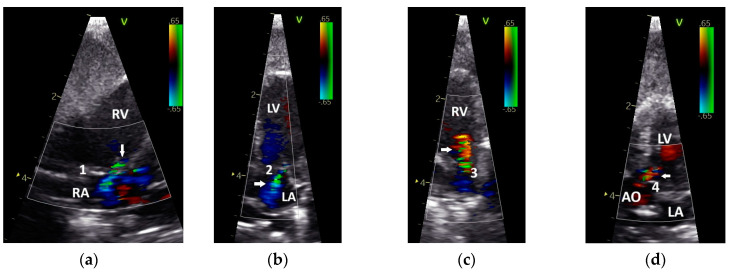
Insufficiencies of the right (a) and left (b) atrioventricular valve and the pulmonary (c) and aortic valve (d) of Congo grey parrots, visualized by colour flow Doppler echocardiography. Arrow: marked insufficiency jet; RV: right ventricle; LV: left ventricle; RA: right atrium; LA: left atrium; AO: aorta; 1: right atrioventricular valve; 2: left atrioventricular valve; 3: pulmonary valve; 4: aortic valve. The colour scale on the right side of the image is calibrated in m s^−1^.
